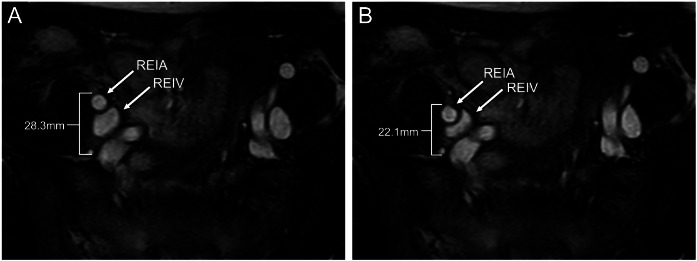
Cine MRI images (A, B) showing strong pulsation of the right external iliac artery, which displaced the artery by up to 6mm and compressed the adjacent external iliac vein. REIA, right external iliac artery; REIV, right external iliac vein. See also Supplementary Video 2.
